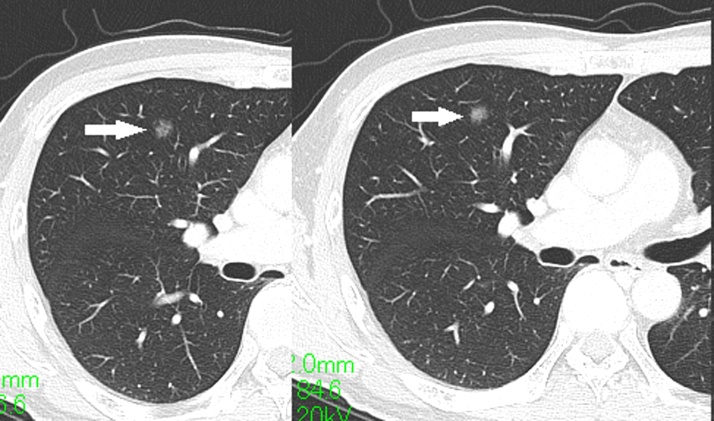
Computed tomography (CT) manifestation of the lung lesion (arrowheads). Chest CT showing a pure ground glass opacity, with a maximum diameter of 8 mm, located in the subpleural area of the right upper lung.
